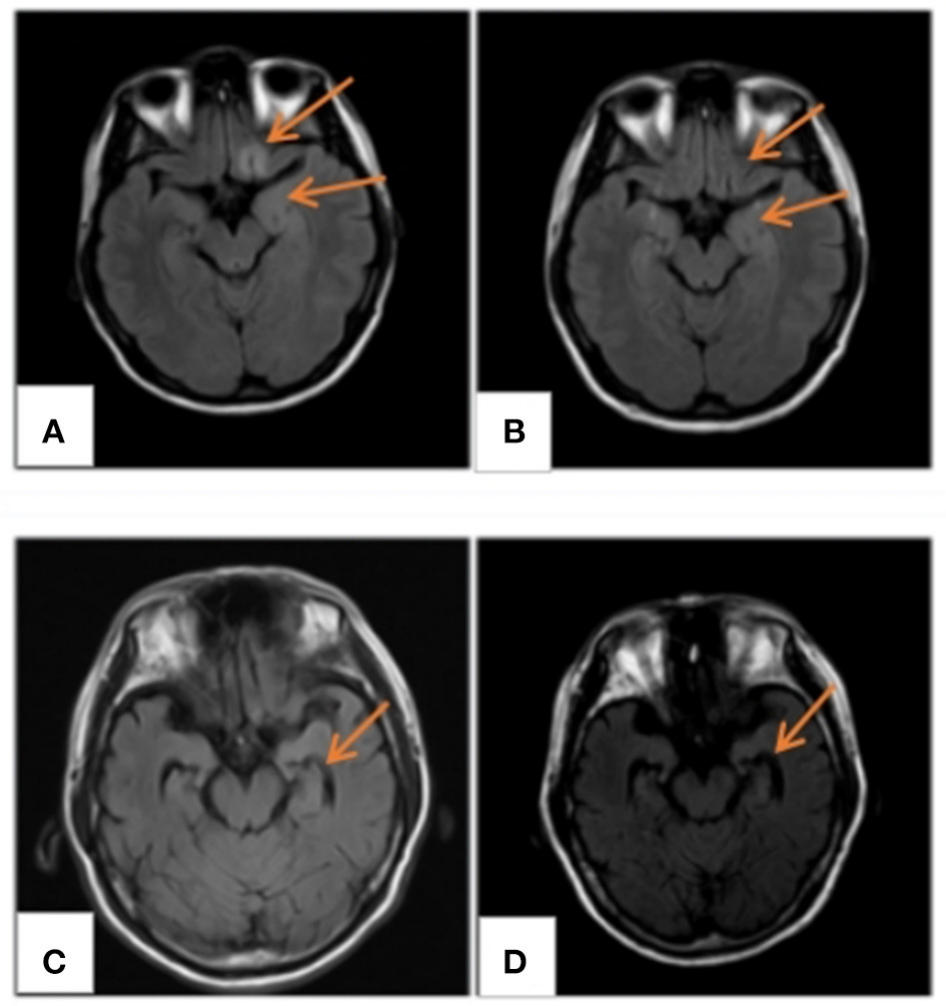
MRI findings. (A) MRI FLAIR/T2 of case 4 displaying abnormal signals in the frontal and insular lobes, (B) normal after 3 months; (C) hippocampal atrophy in case 3; and (D) atrophy development 4 months later. MRI, magnetic resonance imaging; FLAIR/T2, fluid-attenuated inversion recovery.
